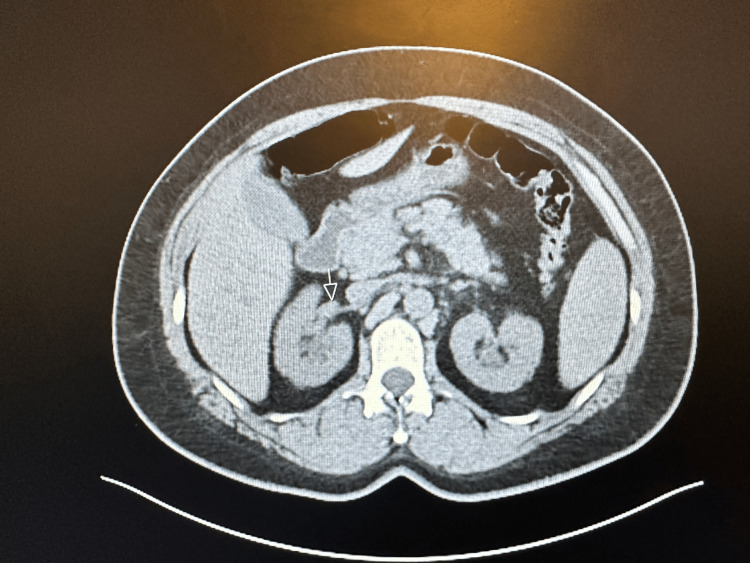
CT-Abdomen showing the right renal pelvis and proximal ureteral thickening.
